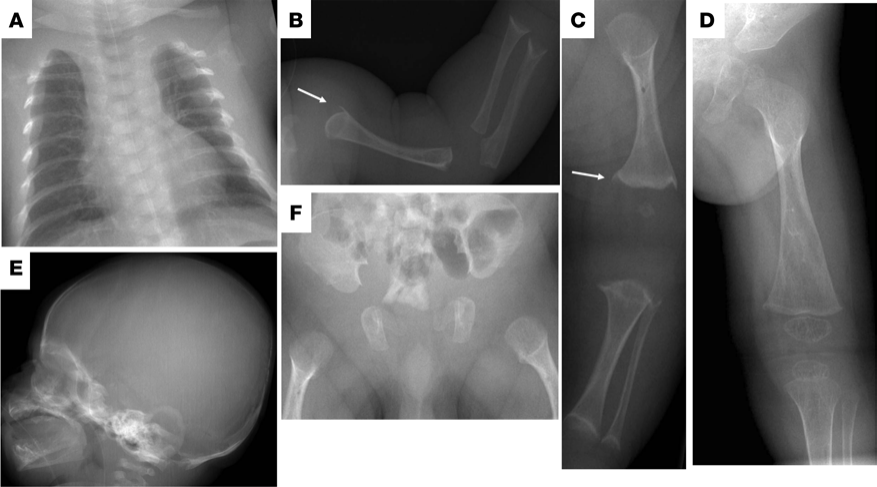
Skeletal characteristics. Radiographs of patient F5-2 with the p.Ile62Ser mutations at age 1–4 months showing thin ribs (A), abnormally short, wide, and poorly mineralized long bones in the arm (B) and leg (C). The proximal humerus and distal femur have abnormal metaphyses with pikes (arrows) reminiscent of those seen in hypophosphatasia. At 4 months, the long bones are poorly mineralized and show marked undertubulation with metaphyseal widening of the distal femur (D). Skull is osteopenic and does not show wormian bones or sclerotic lesions (E). Pelvis shows undermineralization but regular iliac wings and abnormally wide and poorly mineralized proximal femurs (F).
